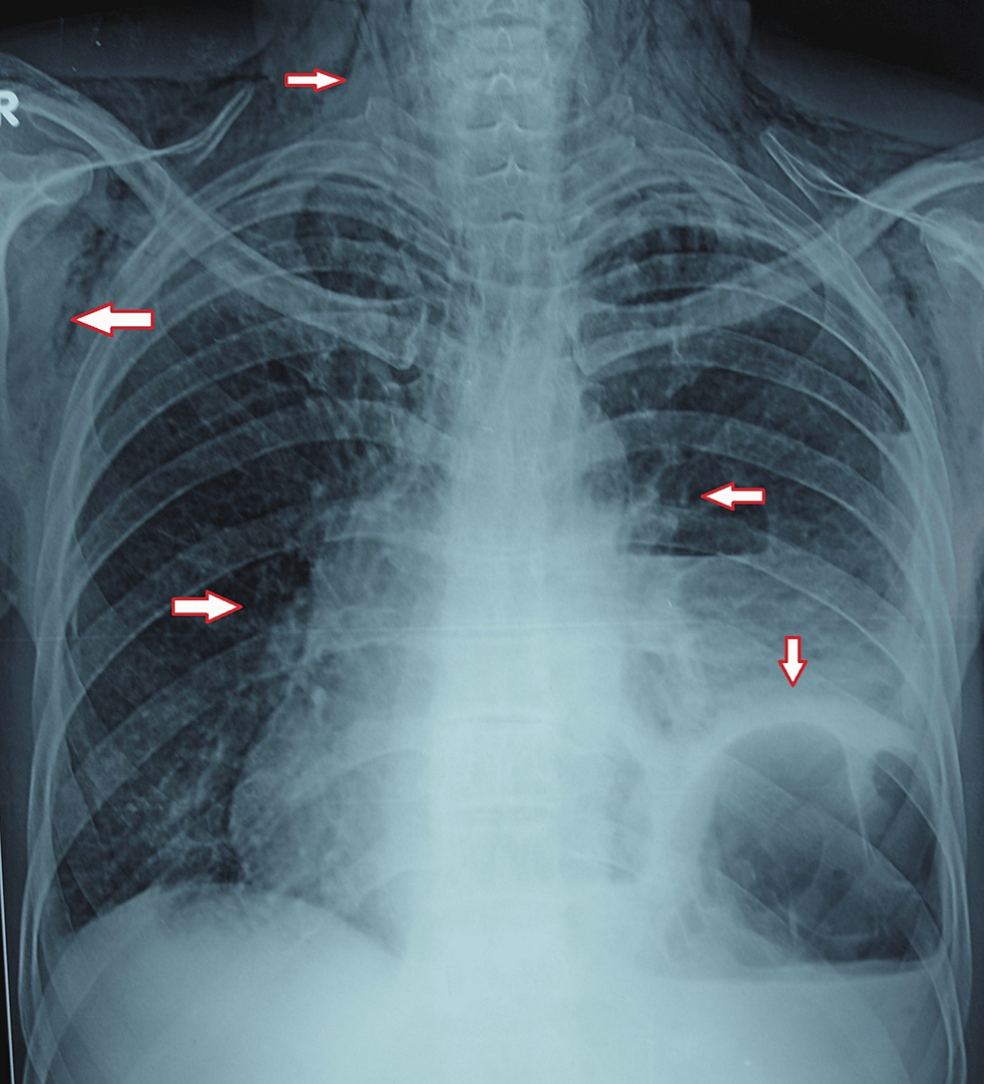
Chest X-ray showing a raised left dome of the diaphragm and air-fluid level with pleural collection along with subcutaneous emphysema in the neck, chest, and axilla.
